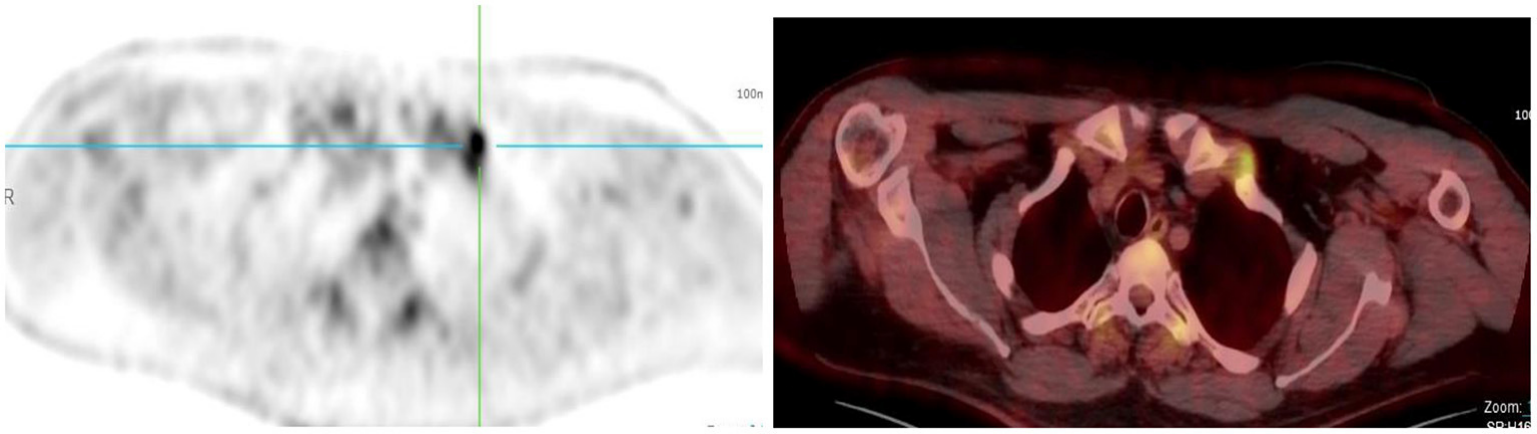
50 year old man with melanoma of the scalp. PET and Combined PET/CT images show intense uptake in the left sternoclavicular joint, which is osteodegenerative.
